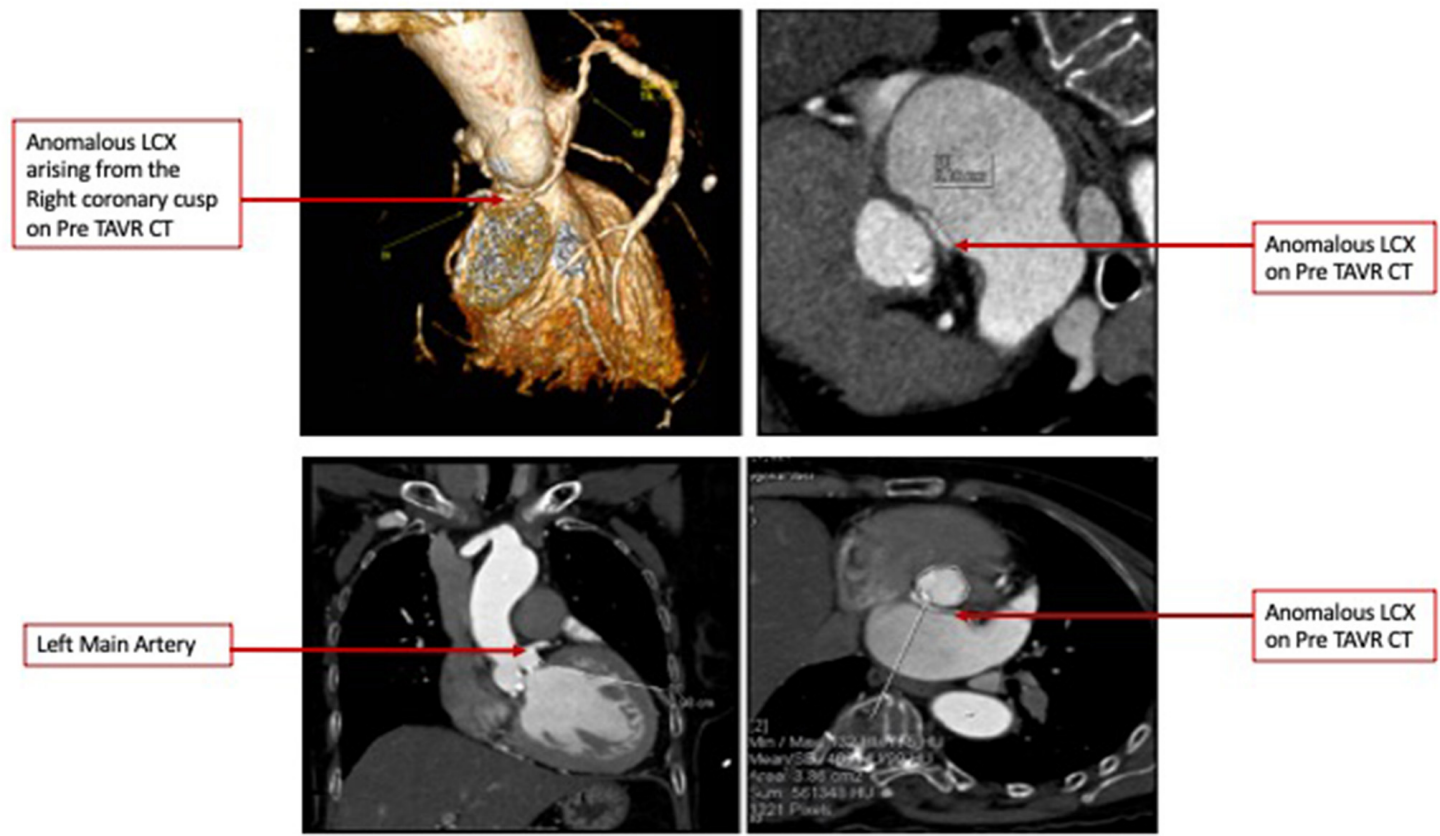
(A) Pre-TAVR Cardiac Computed Tomography 3D volume rendered images demonstrating an anomalous Left Circumflex Artery and Minimal Intensity Projections denoting a course between the Left Atrium and Aorta. (B) Pre-TAVR Cardiac Computed Tomography images demonstrating an Aortic valve annulus of 3.86 cm 2 and Annulus to Left Main distance of 10 mm.
